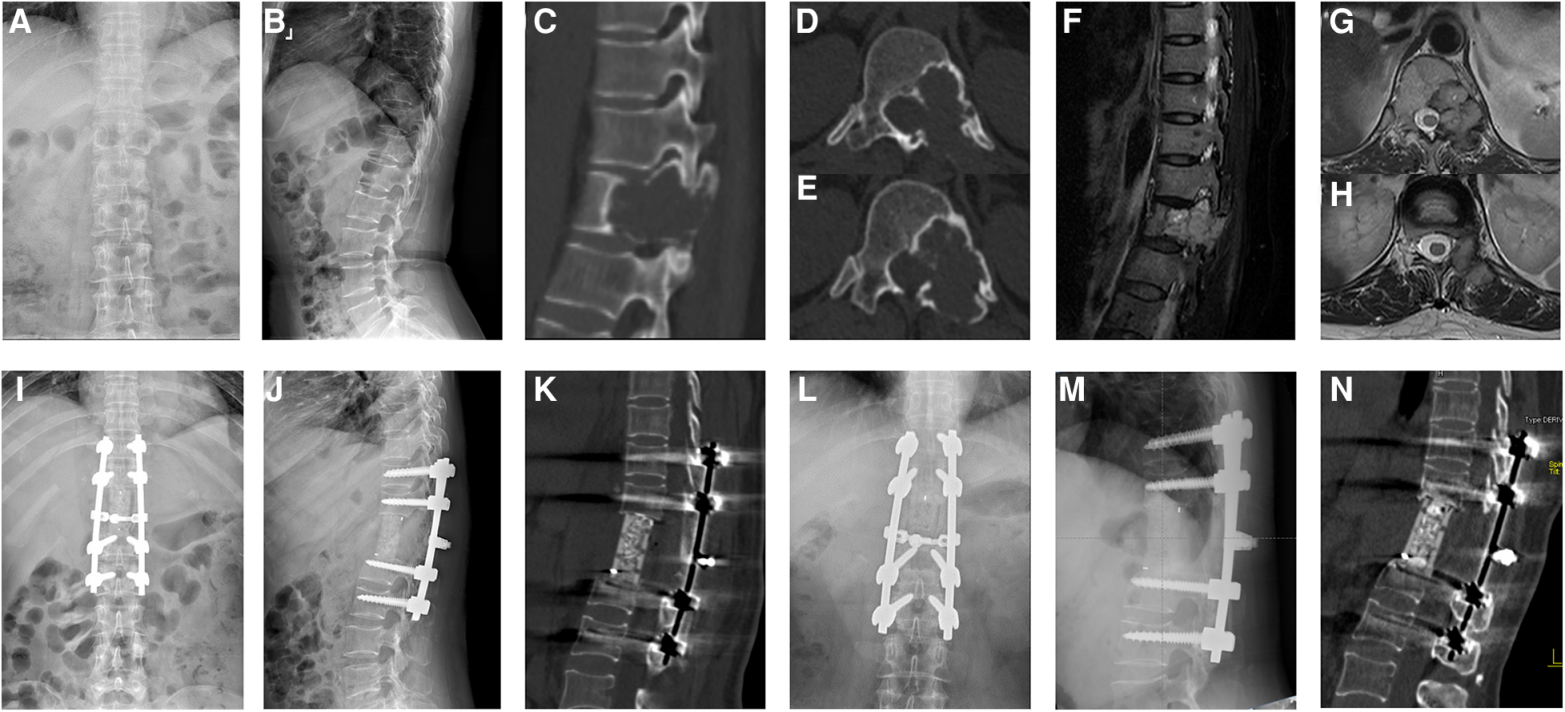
A 50-year-old female patient with a T12 giant cell tumor complicated by neurological deficits. The preoperative anteroposterior (A) and sagittal x-ray (B), sagittal (C) and axial CT (D,E), and sagittal (F) and axial MRI (G,H) scans showed pathological bone destruction of the T12 vertebral body with left intervertebral foramen invasion. The immediately postoperative plain radiographs (I,J) and sagittal CT scan (K) showed corpectomy, screw fusion, and stable spinal alignment. The plain radiographs (L,M) and sagittal CT scan (N) showed the absence of instrumentation failure, tumor recurrence, and cage subsidence at the final follow-up.
